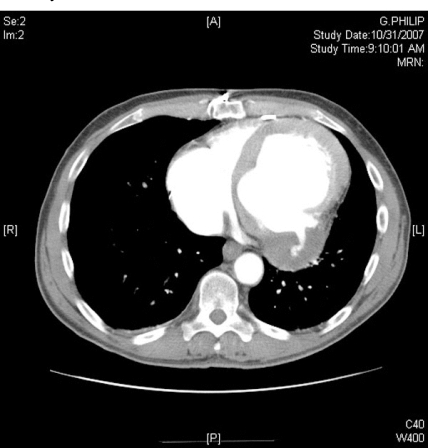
Contrast CT scan demonstrates left ventricular pseudoaneurysm.
